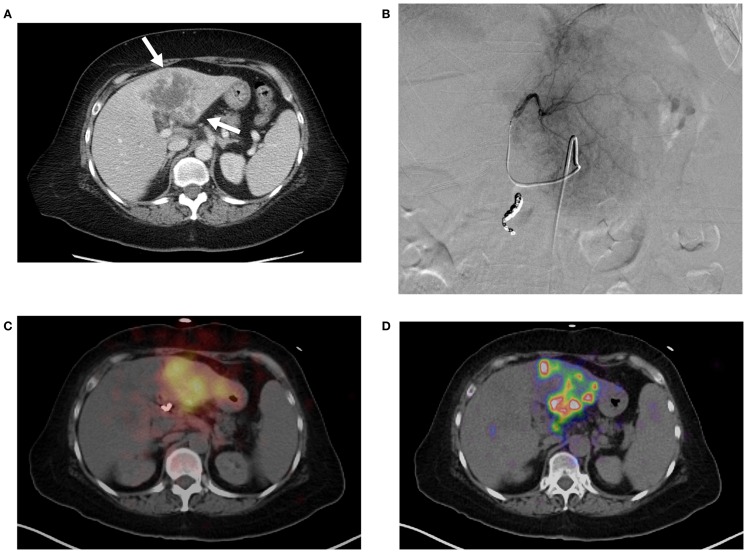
(A) Pre-treatment contrast enhanced CT imaging of metastatic cholangiocarcinoma in the left hepatic lobe. Areas of increased vascularity (arrow) are seen surrounding areas of necrosis. (B) Left hepatic angiogram during infusion of ^90^ Y into left lobe. (C) Bremsstrahlung SPECT following radioembolization demonstrates diffuse activity in the region of the left lobe hepatic neoplasm. (D)
